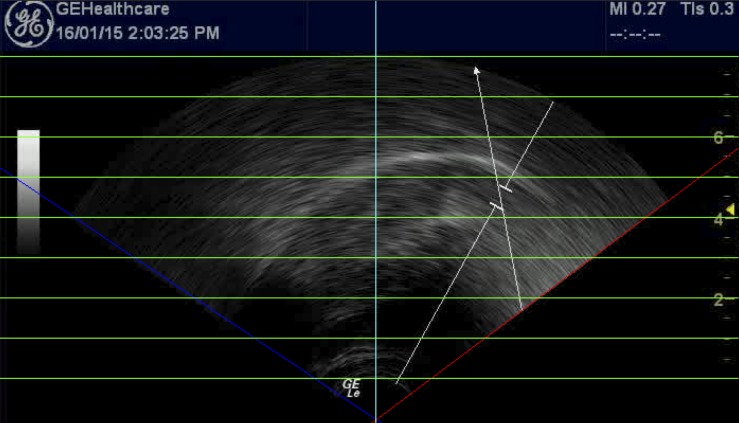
Estimation of the ‘virtual origin’ and pixel scale from a randomly selected ultrasound image by overlaying various lines (slanted blue and red lines delineate the fan-shaped scan area, green horizontal lines replicate the scan depth indicators at right-hand side of the image, and the turquoise vertical line serves as perpendicular reference).
